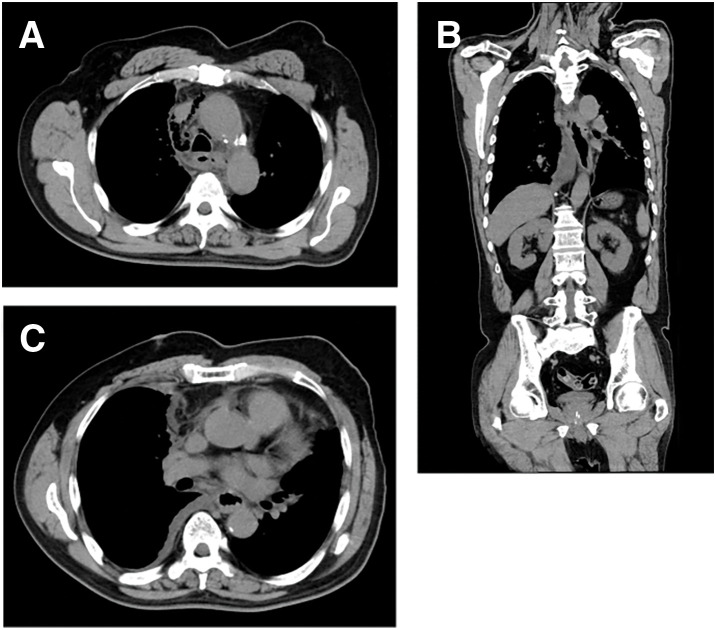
CT findings at the onset of perforation (day 6 of cycle 1). (A, C) Axial CT shows pneumomediastinum and periesophageal fluid collection compatible with esophageal perforation and mediastinitis. (B) Coronal CT demonstrates pneumomediastinum extending cranially toward the anterior mediastinum. Pleural effusion was minimal at this stage.
